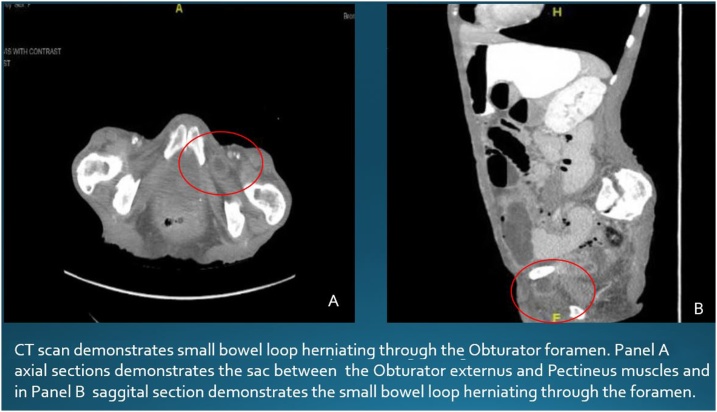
CT scan demonstrates small bowel loop herniating through the Obturator foramen. Panel A axial sections demonstrates the sac between the Obturator externus and Pectineus muscles and in Panel B saggital section demonstrates the small bowel loop herniating through the foramen.
